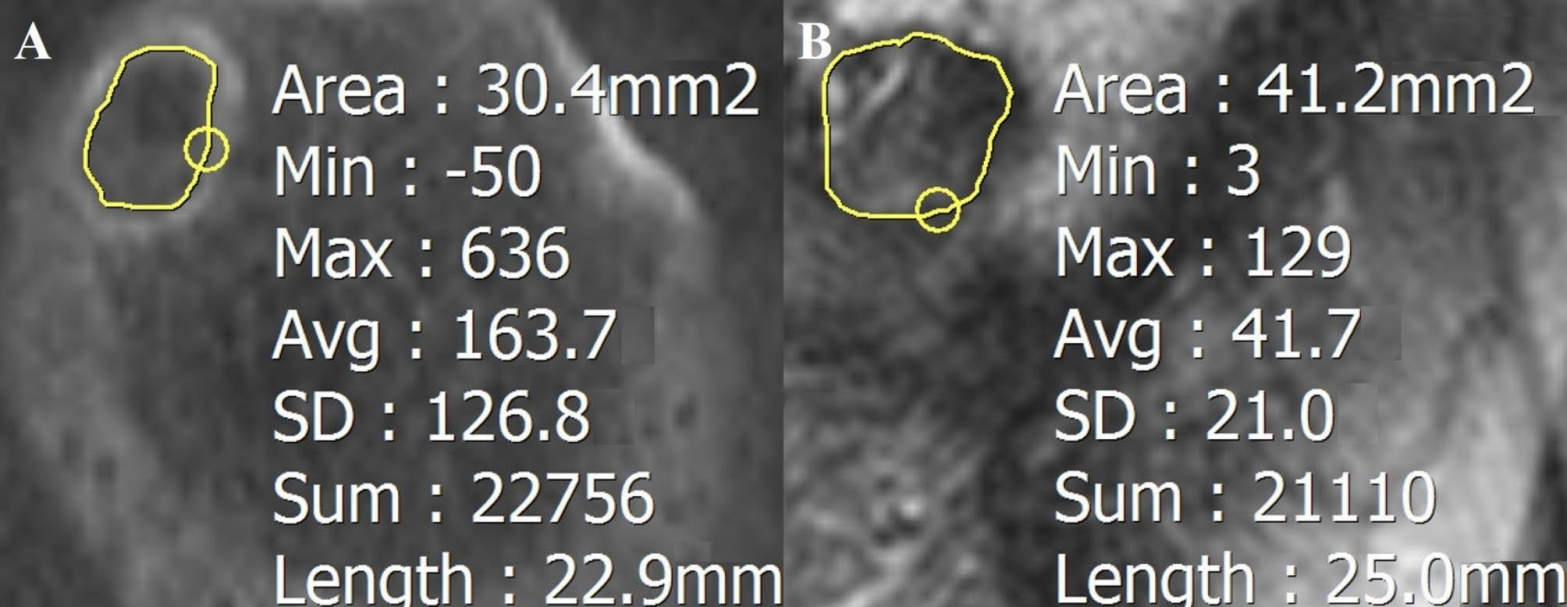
Measurement of the CSA. The lining along the aperture boundary was recorded for the CSA of the femoral tunnel using the freehand technique. (A) CSA on CT at 1 day postoperatively, (B) CSA on MRI at 2 years postoperatively. CSA, cross-sectional area; CT, computed tomography; MRI, magnetic resonance imaging
